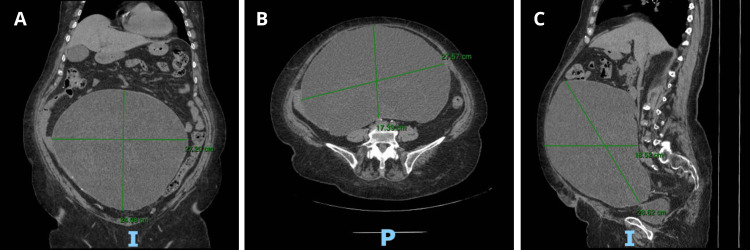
Preoperative abdominal CT scan. The CT scan shows a 27.2 x 24.08 x 18.5 cm abdominal cyst with a probable mesenteric origin with almost complete occupation of the abdominal cavity. (A) Coronal view. (B) Axial view. (C) Sagittal view.
